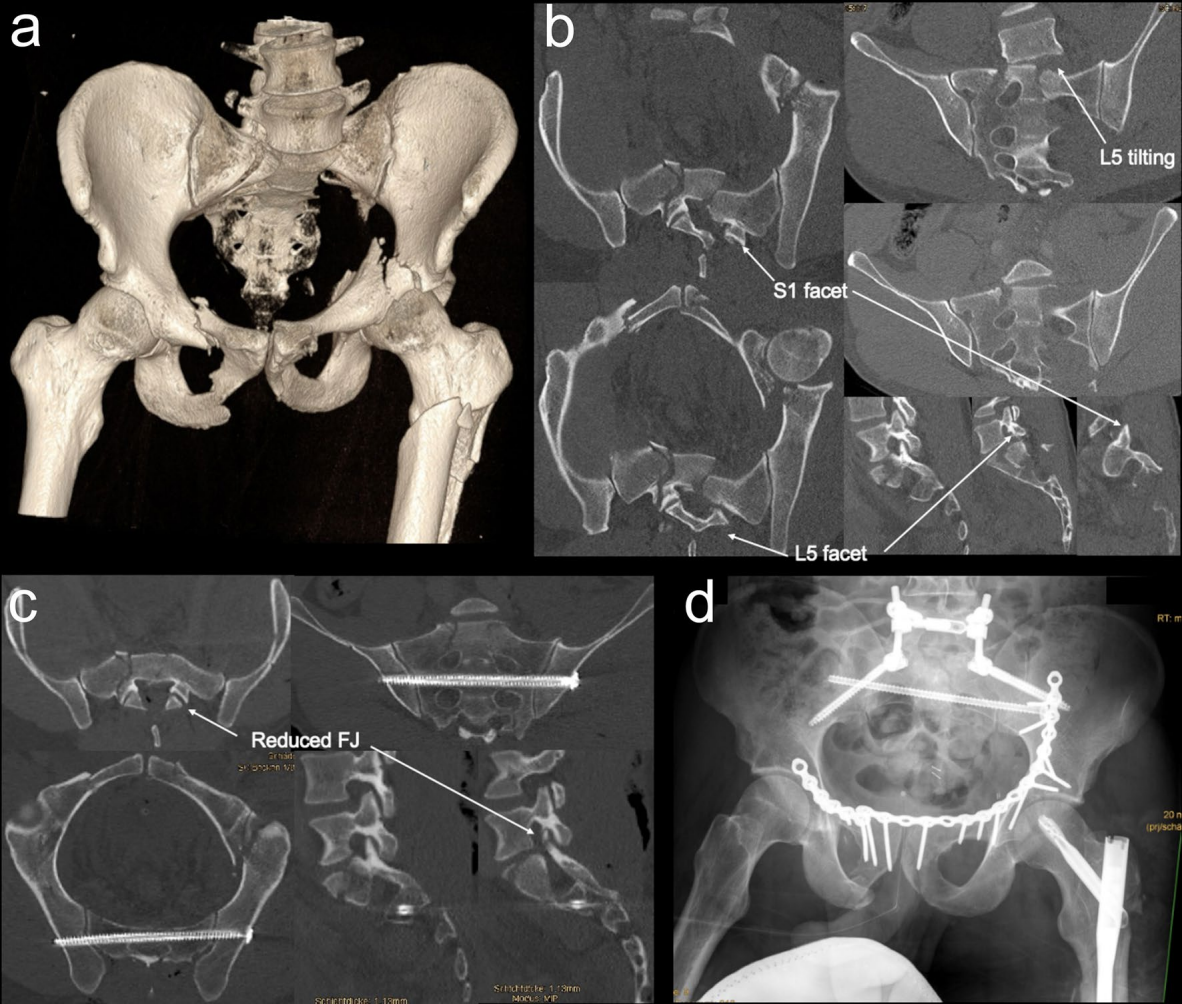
Bilateral vertical sacral fracture without a transverse fracture component (BVSF; Type 4 TLSI). A 37-year-old male polytrauma patient fell from a height of 8 m in a paragliding accident and presented with left-sided L5 and S1 nerve root dysfunction. a 3D CT reconstruction showing a BVSF associated with an anterior pelvic ring injury and a left-sided acetabular fracture. b Multiplanar CT reconstructions demonstrating left-sided lumbosacral facet joint dislocation and coronal tilting of the L5 vertebral body. c CT images obtained after immediate open reduction, decompression, and dural repair via a posterior midline approach, followed by transsacral screw fixation in S2. d AP radiograph after staged anterior pelvic ring and acetabular fracture fixation, as well as additional L5-ilium instrumentation and fusion performed on the following day
